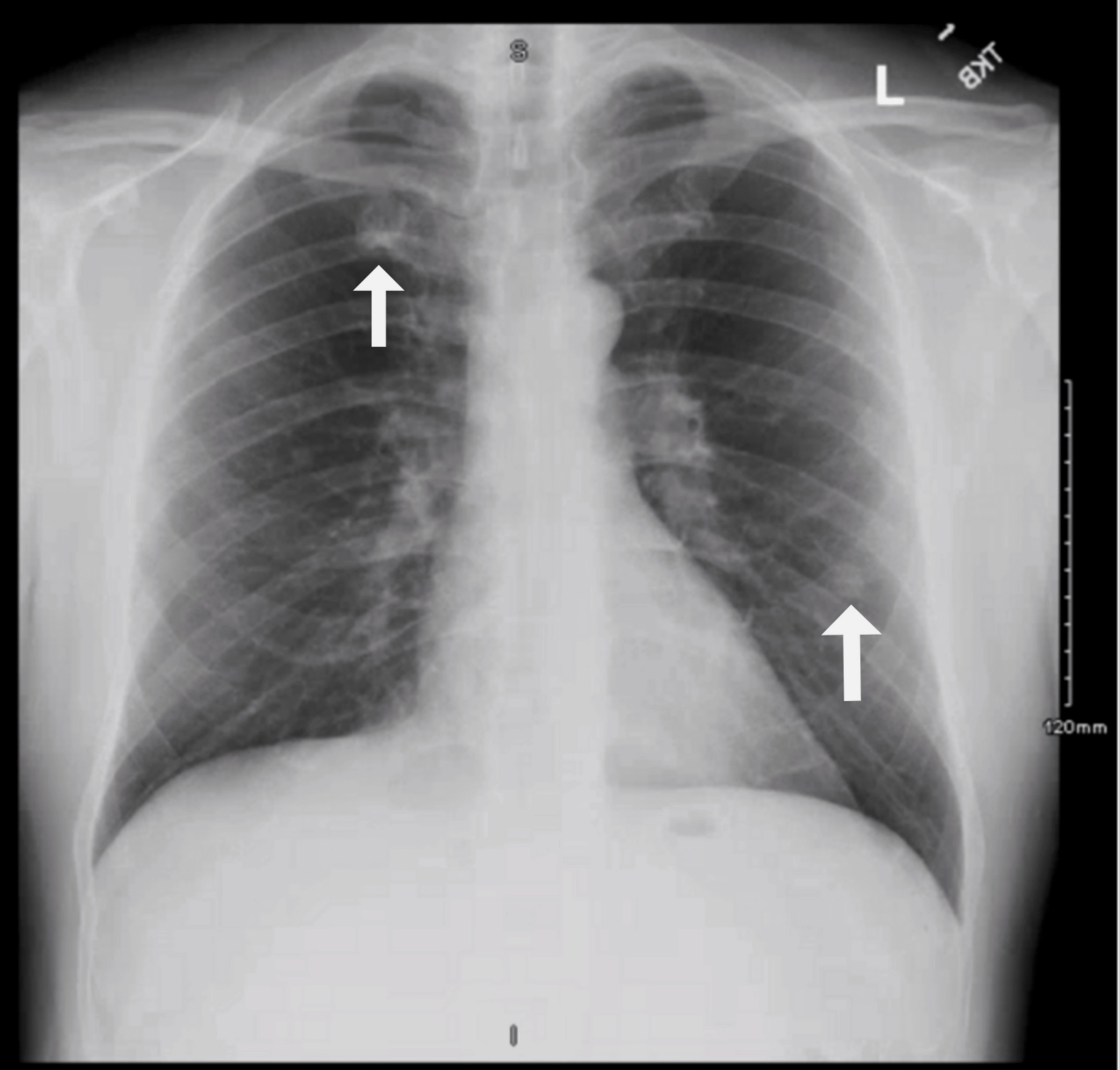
Chest X-ray demonstrating left-sided infrahilar region and right upper lobe nodules
